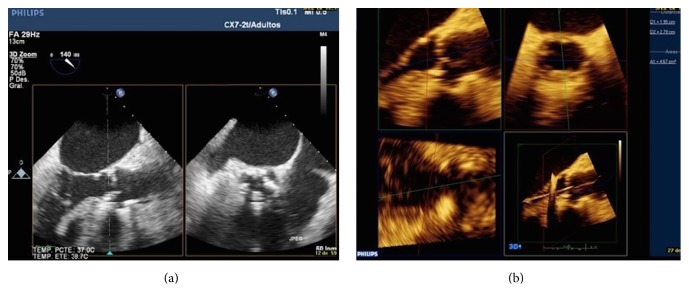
AVA calculation with 2D (a) and 3D transesophageal echocardiography (b).
